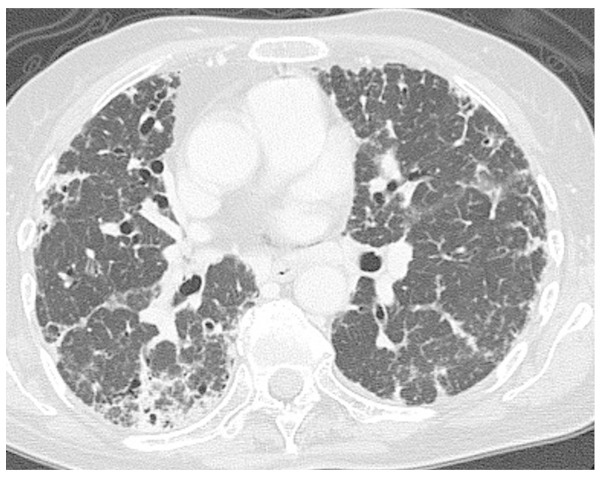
Chest computed tomography scan showing lung fibrosis at the time of diagnosis of progressive systemic sclerosis.
